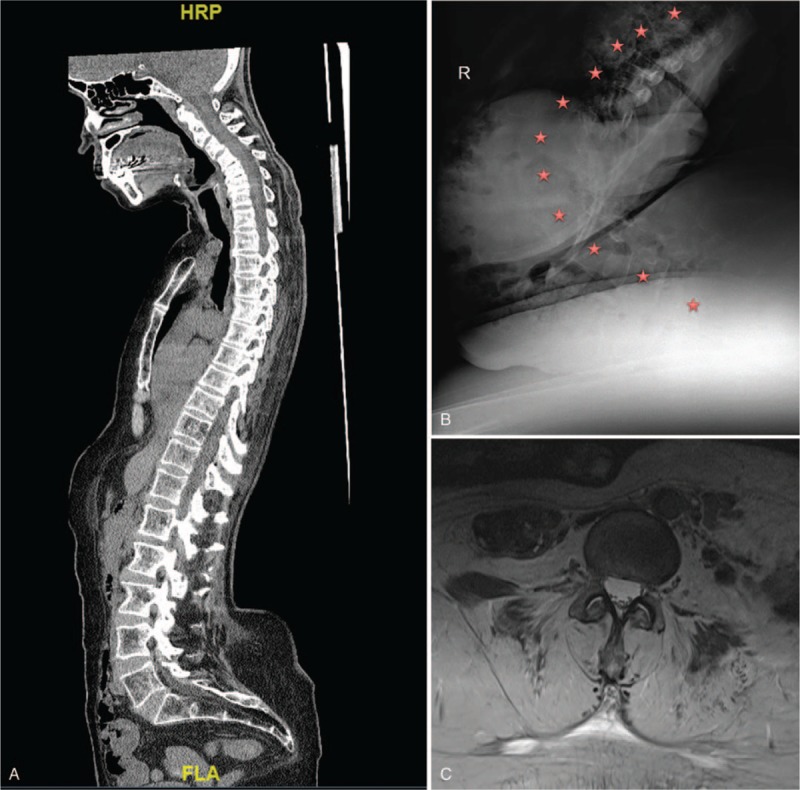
Her radiologic assessment in supine revealed that her deformities were completely flexible, as shown in the sagittal plane computerized tomography scan (A). Her deformity was obvious on lateral lumbar X-ray while sitting. Vertebrae corpuses are marked in red (B). Preoperative MRI revealed severe paraspinal muscle atrophy (C).
